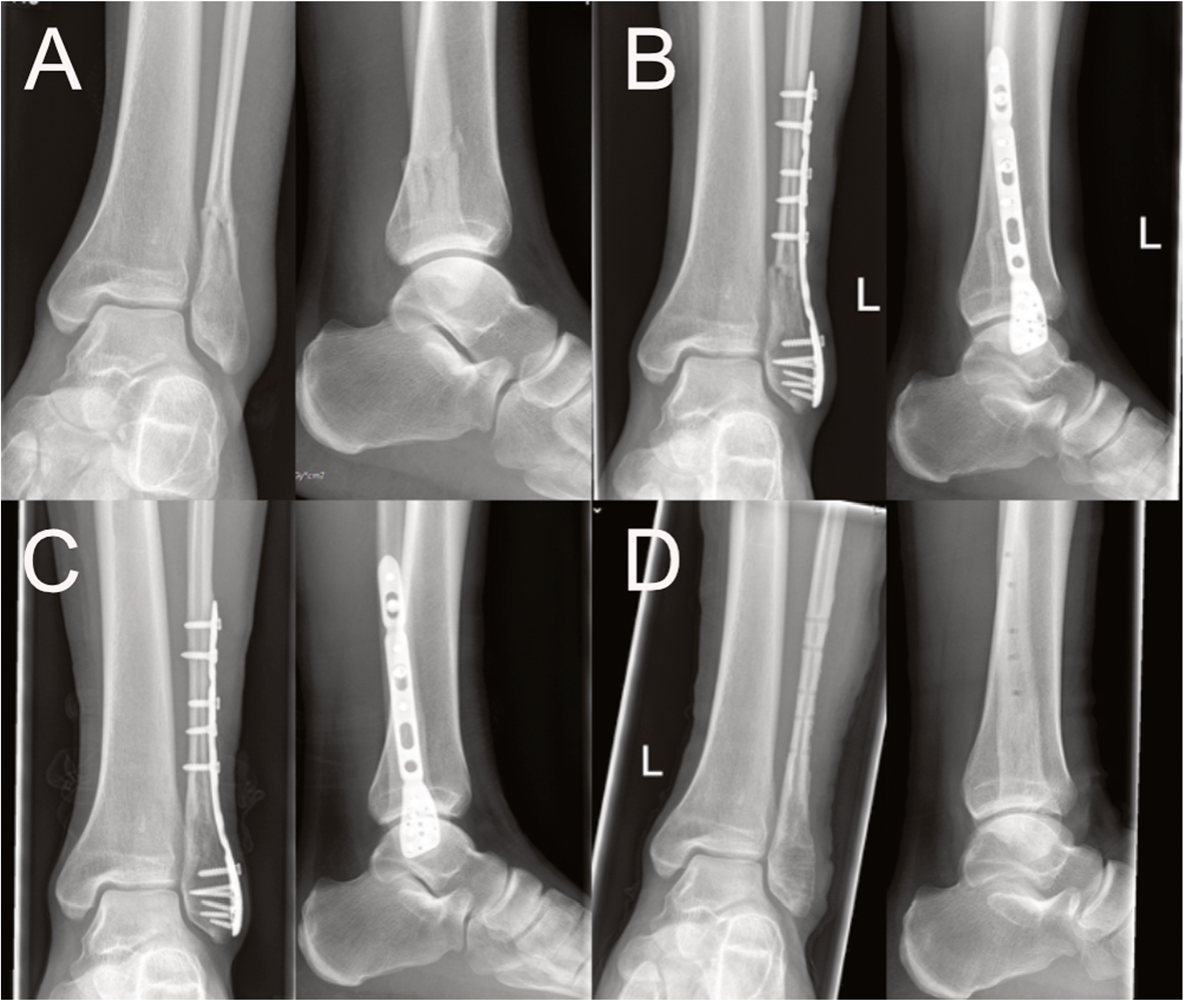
Radiograph of an 18-year-old male patient suffering from an AO type 44B1.1 fracture in two planes (a). The patient was treated with the Newclip Technics® Active ankle system. Figure b shows the radiographic follow-up 6 weeks after surgery with image c) presenting the follow-up after 12 months. Finally image d) demonstrates the status after hardware removal (13 months postop)
